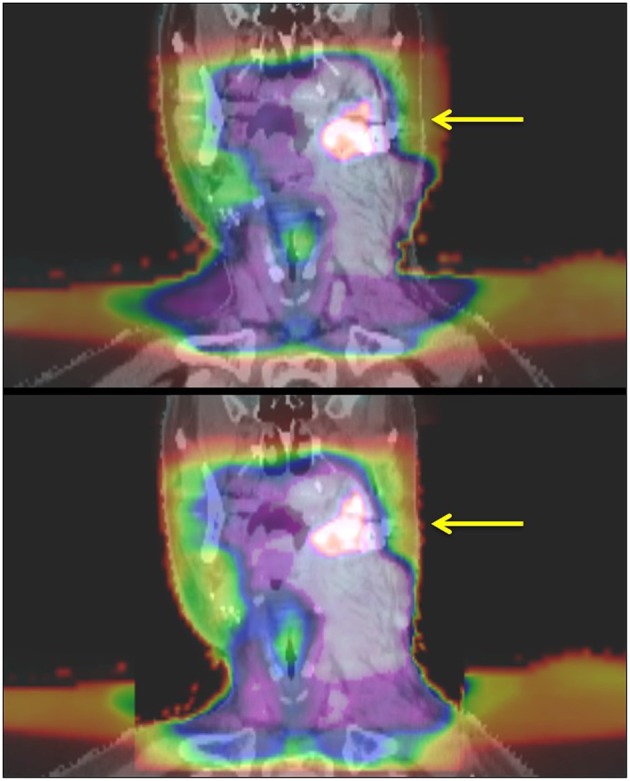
Deformable registration of initially delivered radiation doses onto retreatment planning CT. The yellow arrow highlights the PET-positive tumor. The top panel shows fusion of doses onto CT based on anatomical landmarks before deformable registration. The bottom panel shows deformably registered images with better delineation of doses delivered to normal tissue structures.
